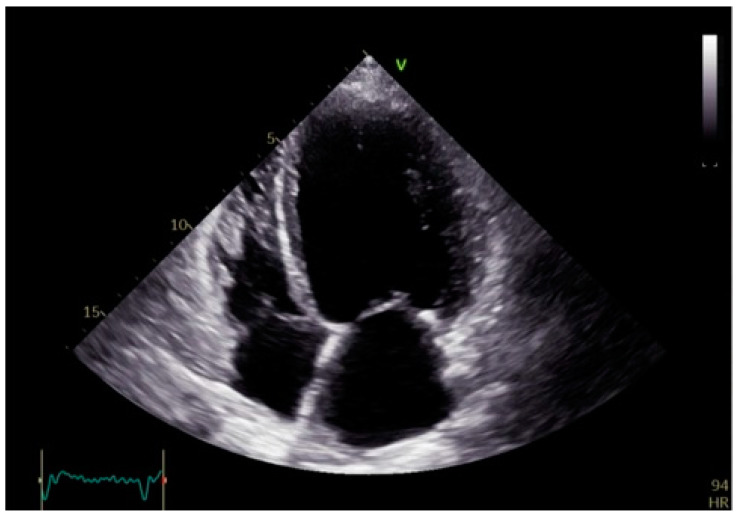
Apical four-chamber echocardiographic view showing dilated left ventricle with a fibrotic interventricular septum, characterized by increased echogenicity and reduced mobility, suggestive of structural remodeling.
